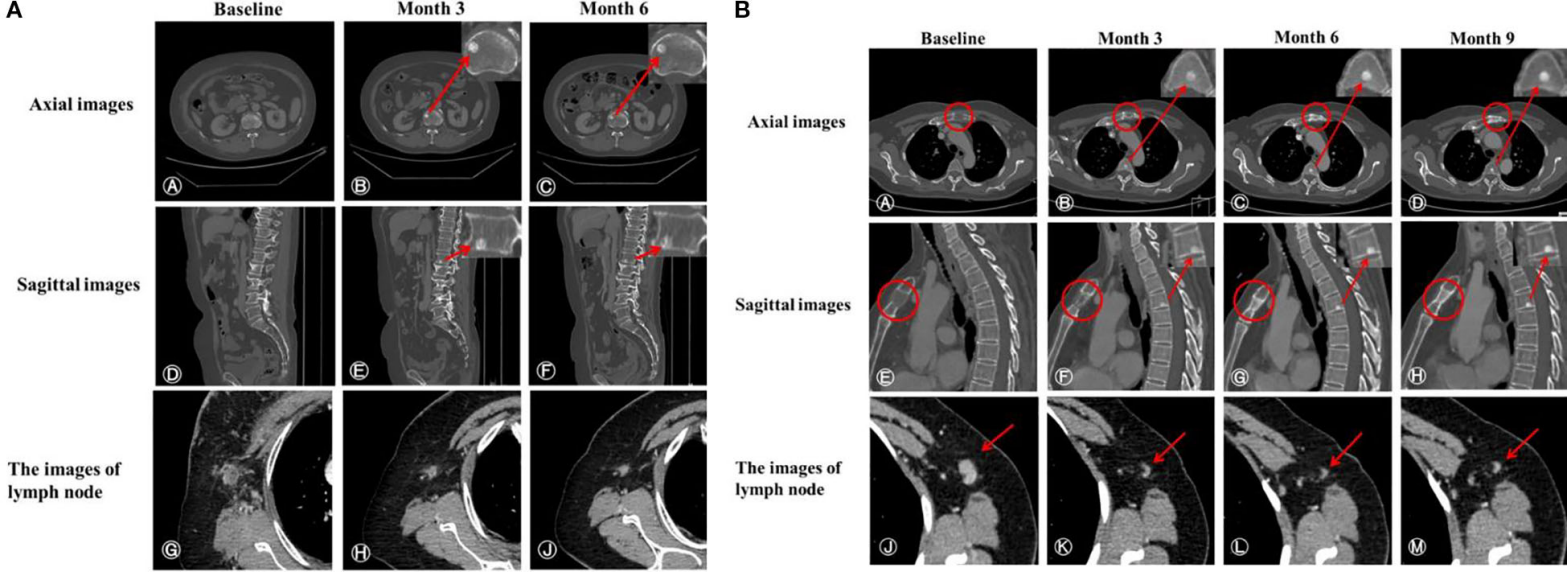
(A) CT scans of a patient demonstrating bone pseudoprogression. (A, D) Pretreatment axial and sagittal CT images (bone window); (B, E) Three-month follow-up reveals a new, well-demarcated, round high-density lesion at the first lumbar vertebra’s inferior edge; (C, F) Six-month evaluation shows a sclerotic nodule of similar extent with slightly decreased density; (G) Pre-treatment image of an enlarged right axillary lymph node (short diameter 16 mm) with heterogeneous density and indistinct fat margins; (H, J) Post-treatment images at three months (short diameter 9 mm) and six months (short diameter 7 mm) demonstrate continuous lymph node regression. (B) CT scans of a patient with previous osteolytic metastases demonstrating bone pseudoprogression. (A, E) Pre-treatment axial and sagittal bone window images reveal osteolytic metastasis in the sternum. (B, F) Three months post-treatment, CT images show a new sclerotic nodule at the lower edge of T4 with clear borders and sclerosis of the original osteolytic metastasis in the sternum. (C, G) Six months post-treatment, the sclerotic nodule and the sternal osteolytic metastasis show increased sclerosis and repaired. (D, H) Nine months post-treatment, lesion stabilization is observed. (J) Pre-treatment left axillary lymph node enlargement (short diameter 10 mm). (K–M) Post-treatment regression of left axillary lymph nodes (short diameter 3 mm).
